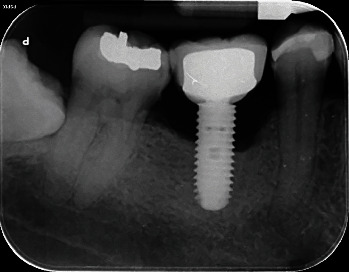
Radiograph of one of the included implants with peri-implantitis: the most common defect conformation in the present study was crateriform, with both intraosseous and horizontal components.
